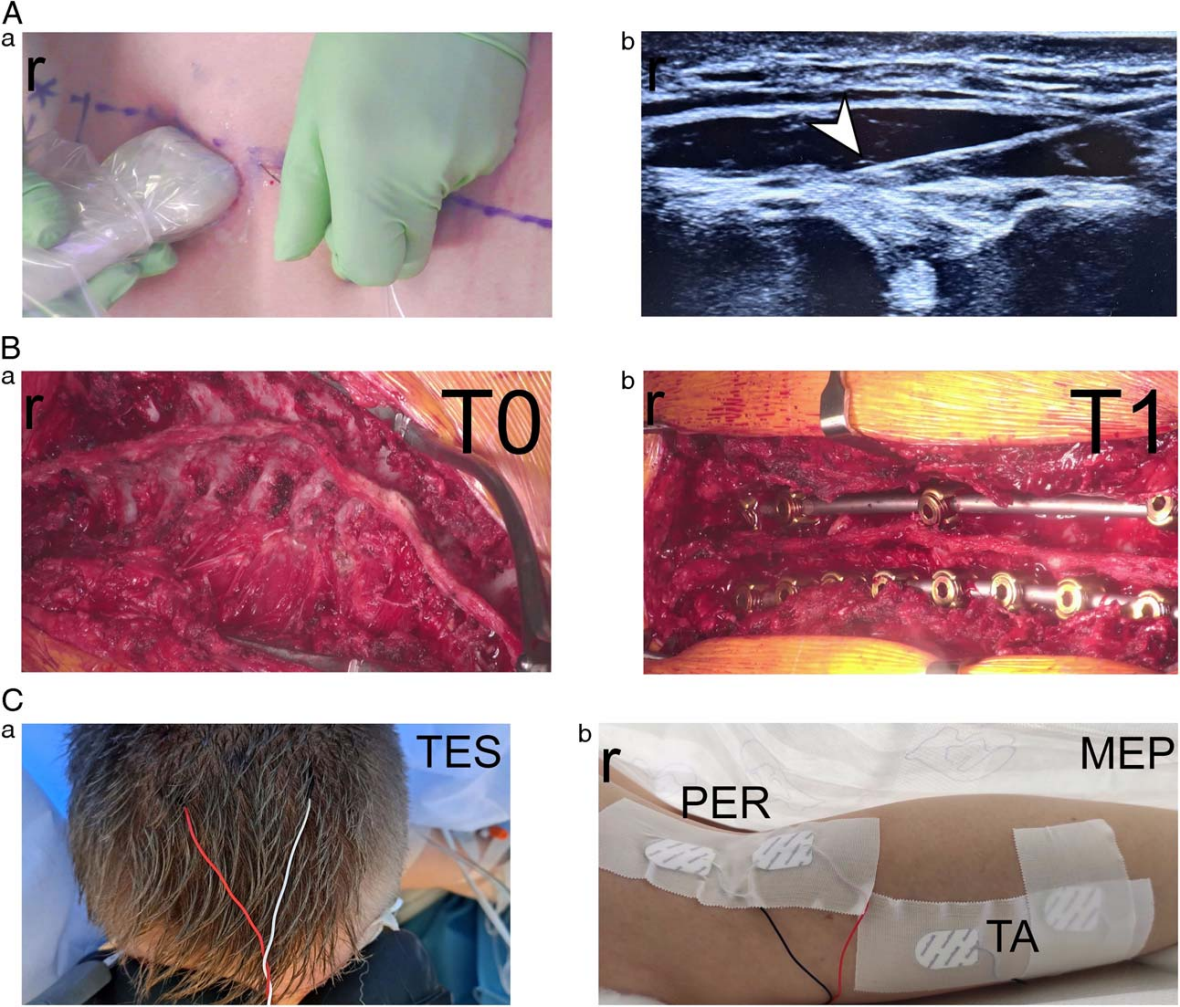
A, EPSB technique under ultrasonography control (a), the arrowhead indicates the location of the needle tip (b). B, Camera pictures of the surgical field before (a, T0) and after (b, T1) the implantation of the scoliosis corrective instrumentation with transpedicular screws and rods. C, Principles of the transcranial electrical stimulation (a, TES) with needle electrodes implanted over the scalp skin and surface electrodes placed bilaterally on tibialis anterior muscles (b, TA) and over the peroneal nerves (b, PER) for motor-evoked potential (MEP) recordings are presented in Figure 3. r indicates rostral direction.
